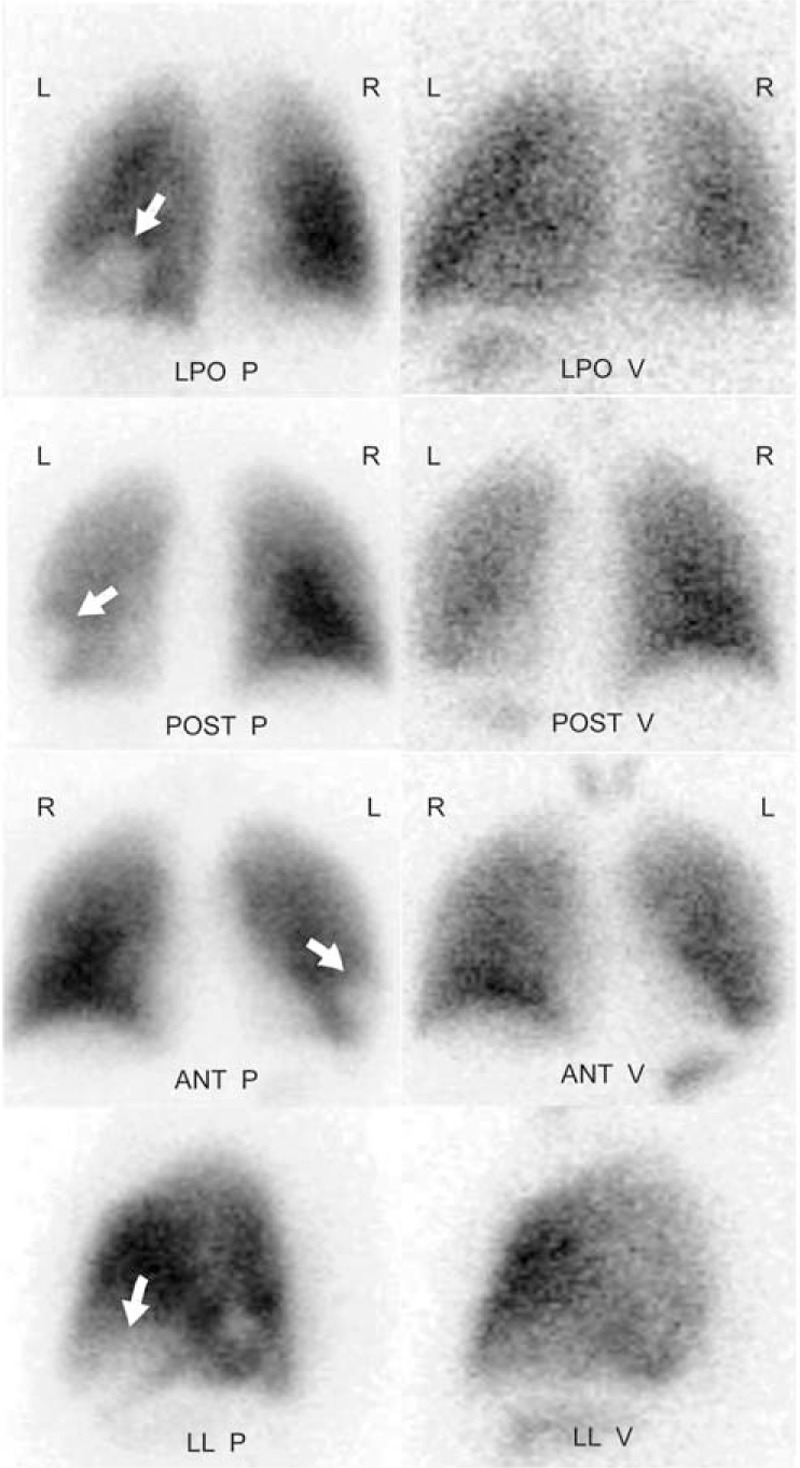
V/Q scan for PVS patient. Case 3: radionuclide V/Q scintigraphy images show a severe reduction in perfusion to the left lobe, and slight reduction in ventilation, without obvious defects. The V/Q mismatched. PVS = pulmonary vein stenosis, V/Q = ventilation–perfusion.
